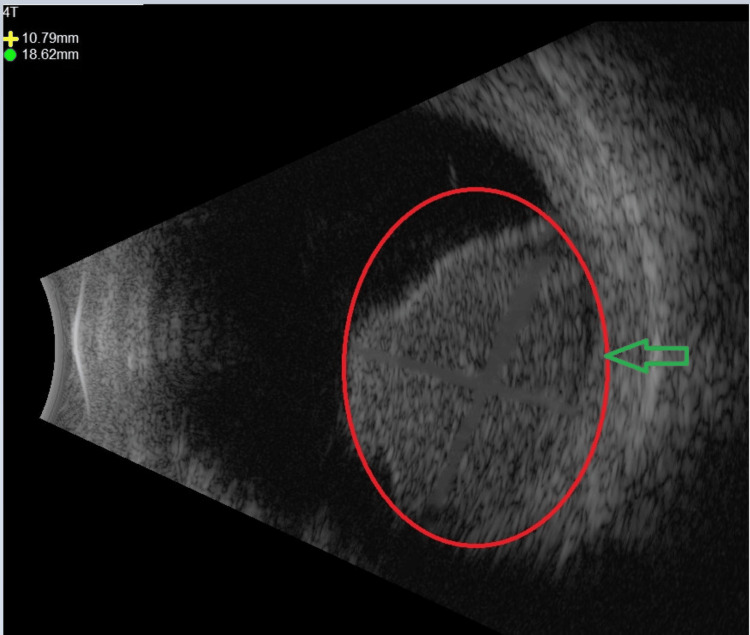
B-scan ultrasonography, at 4 o’clock transverse orientation, showing a classic collar-button appearance.
